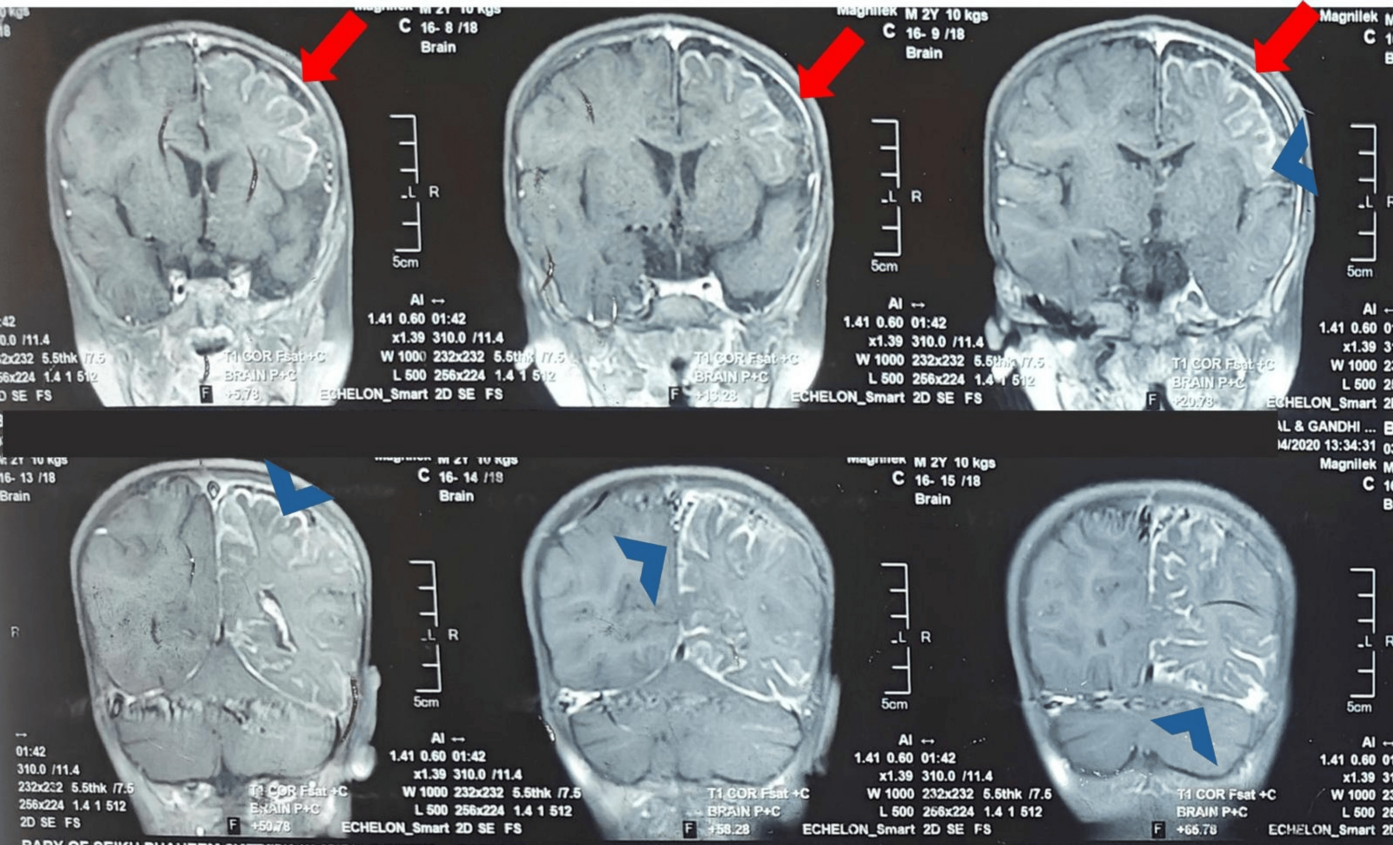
Contrast-enhanced coronal MRI brain images showing leptomeningeal enhancement (blue arrowheads) and underlying cortical atrophy (red arrows) in the left cerebral hemisphere, characteristic of Sturge-Weber syndrome
